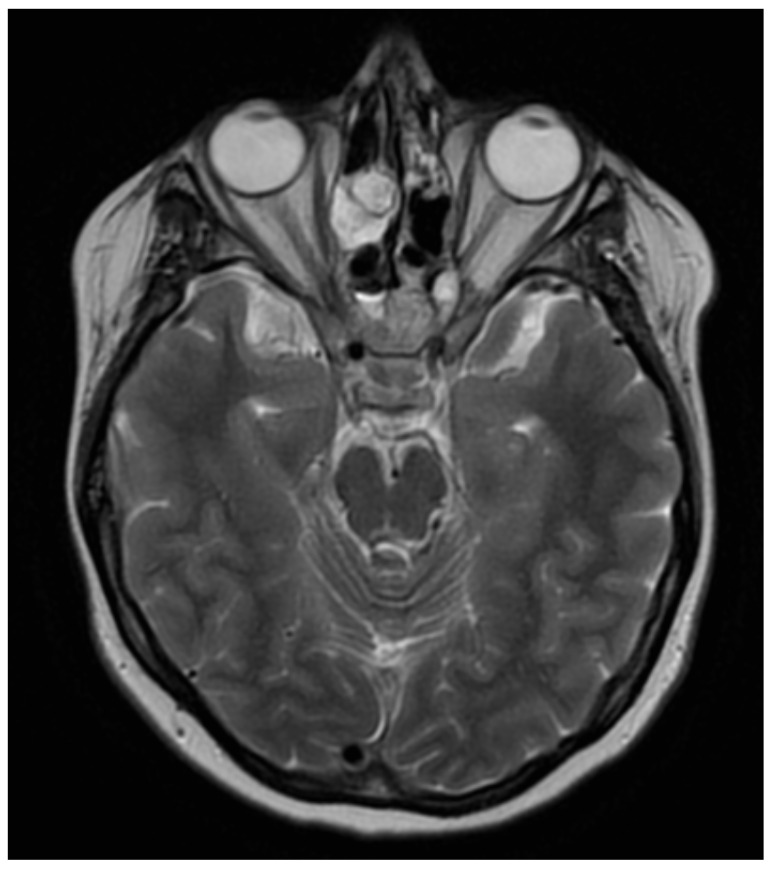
MRI of coronal plane with a massive lesion in the right ethmoid sinus cavity.
